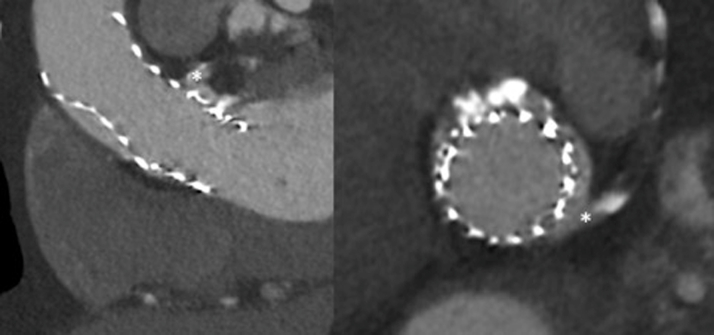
CT Scan, Multiplanar Reconstructions The images show the overlap between the Sapien valve and the Evolut skirt, in front of the left main artery (∗). The valve-to–left main coronary ostium distance measures approximately 3 mm, and the valve-to-sinus junction is virtually absent in the left sinus. CT = computed tomography.
